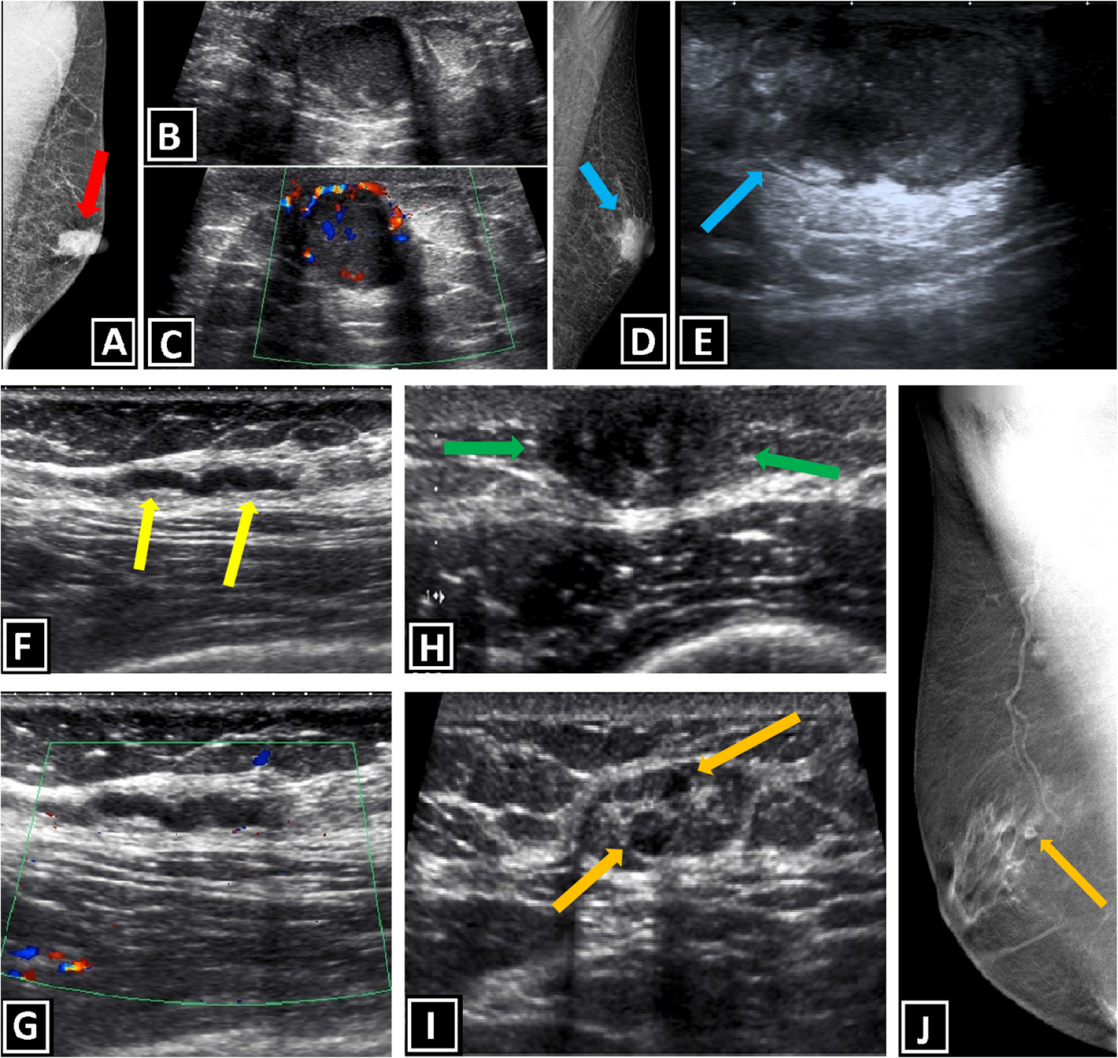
Benign non-neoplastic breast lesions. a–c Fat necrosis and periductal mastitis. Mammography of a 44-year-old man with the complaint of left retroareolar pain shows circumscribed retroareolar lesion with high density (red arrow, a). Well-defined, round hypoechoic lesion with internal and peripheral vascularity is seen in US (b, c). Biopsy reveals the diagnosis. d, e Subareolar abscess secondary to ruptured folliculitis. Mammography of 64-year-old man with retroareolar mass and skin erythema shows subareolar lesion with spicular margins and high density (blue arrow, d). US for exclusion of malignancy reveals heterogeneous, hypoechoic mass which has cystic component with internal echogenities and irregular walls (blue arrow, e). Increased vascularity in surrounding tissues is also present (not shown). Pathologic examination of mastectomy material reveals the diagnosis. f, g Mondor’s disease. US and Doppler US examinations of 32-year-old patient with localized right breast pain show “beaded tubular vascular structure with irregular walls and minimally increased peripheral echogenicity, without apparent blood flow” at the symptomatic area (yellow arrows, f). h Duct hyperplasia. Retroareolar well-defined hypoechoic lesion is shown in a 41-year-old man with nipple discharge (green arrows). i Two small intramammary lymph nodes with circumscribed margin and round shape are shown (orange arrows). j Breast tomosynthesis of another patient reveals gynecomastia accompanied by intramammarian lymph node with round shape and radiolucent fatty hilus (orange arrow)
